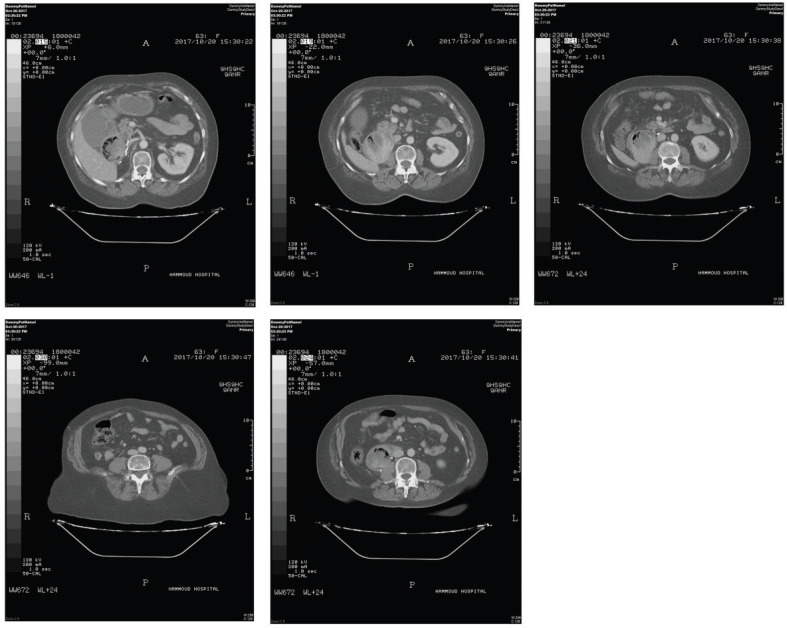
CT scan showing the duodenal mass separated from the recurrence in the nephrectomy fossa.
